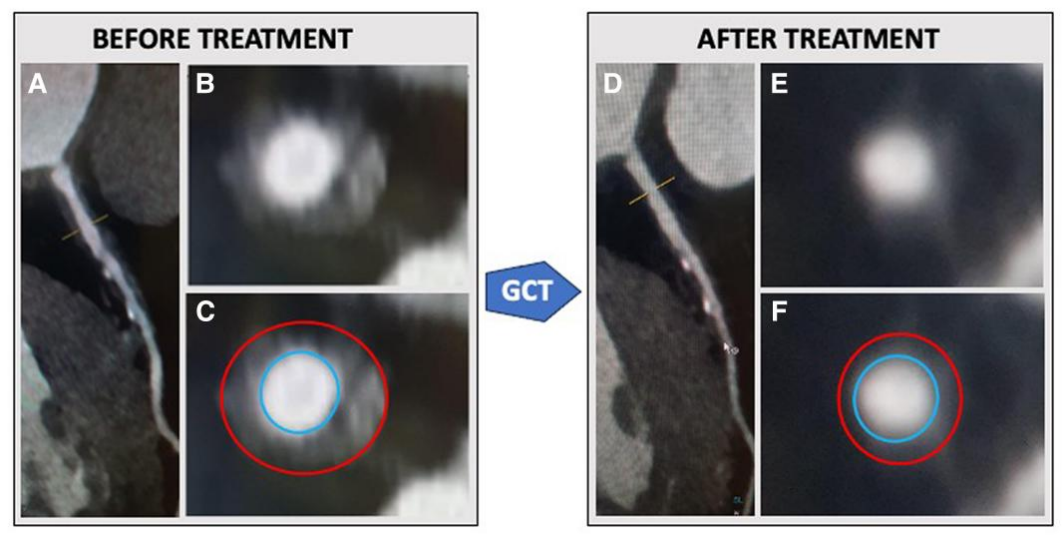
Regression of circumferential thickening of the left main and left anterior descending coronary arteries before (*A–C*) and after (*D–F*) glucocorticoids in cardiac computed tomography angiography. Unmarked (*B*, *E*) and marked (*C*, *F*) cross-sectional images of the left descending coronary artery corresponding to the yellow line level of the curved multiplanar reconstruction (*A*, *D*). In the marked images, the circumferential thickening is visualized between the red line (artery outerwall) and blue line (artery lumen). GCT, glucocorticoid.
